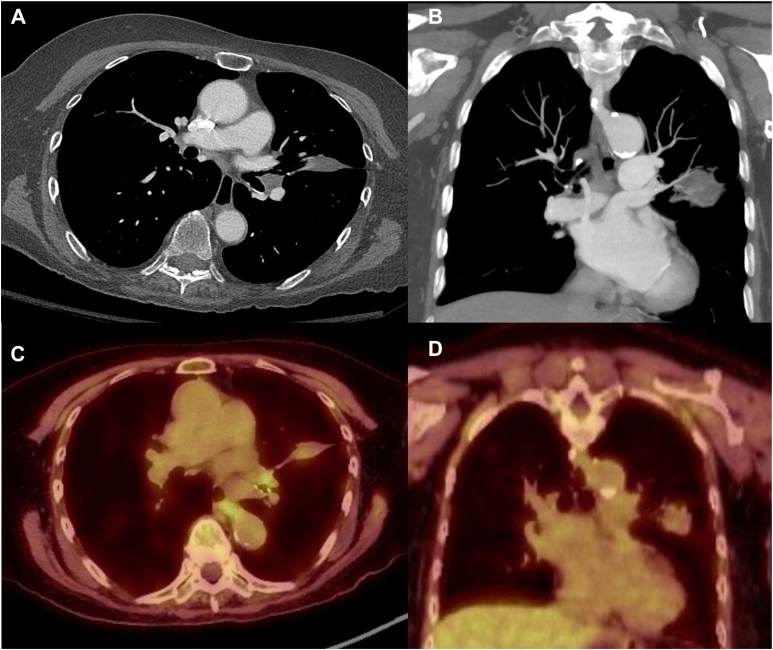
(A, C) Transverse and (B, D) coronal cross-sections on computed tomography and positron emission tomography imaging, respectively, demonstrate the patient’s left-sided non-small cell lung cancer.
